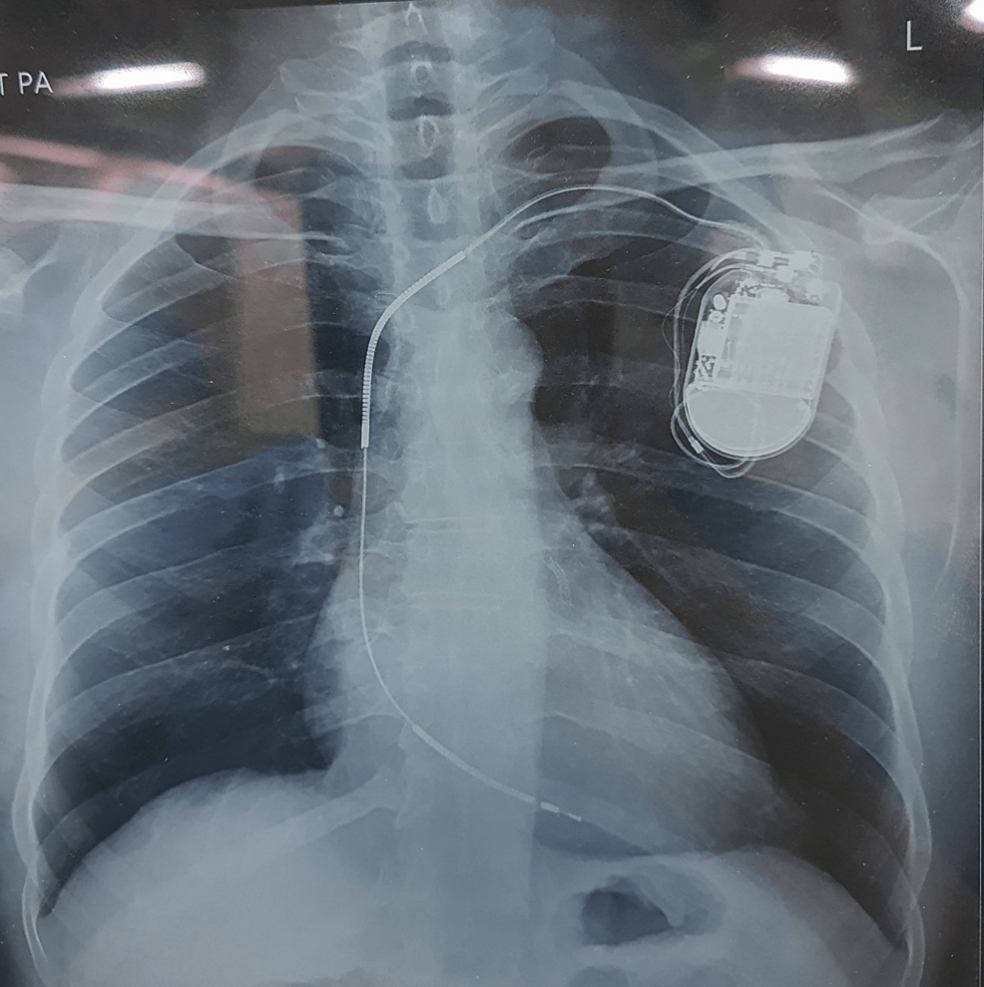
Chest X-Ray of the patient with AICD in situ
